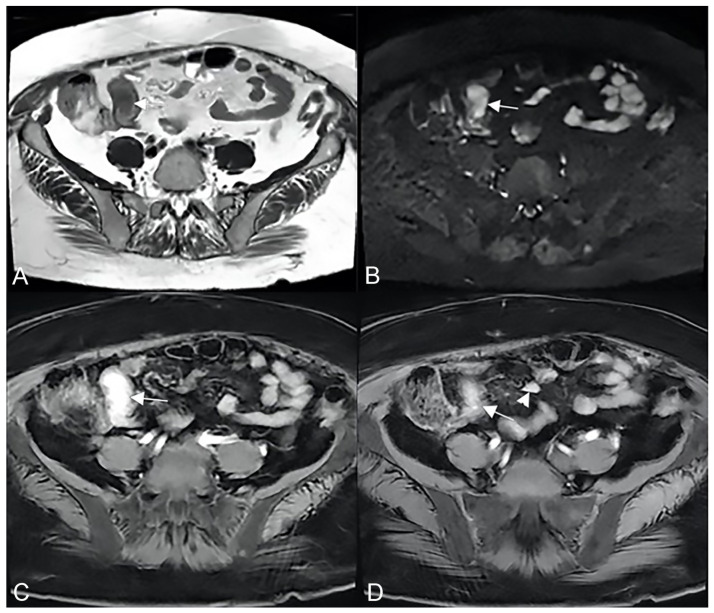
Example of NET in a 56-year-old female patient with abdominal pain and weight loss. (A) Axial T2-weighted, (B) DWI, and (C,D) T1-weighted after gadolinium injection images show focal asymmetric wall thickening of the distal ileum (arrow), with restriction in DWI (arrow in (B)) and marked enhancement in the arterial phase (arrow in (C,D)); an hypervascular mesenteric enlarged lymph node is also evident (arrowhead in (D)).
